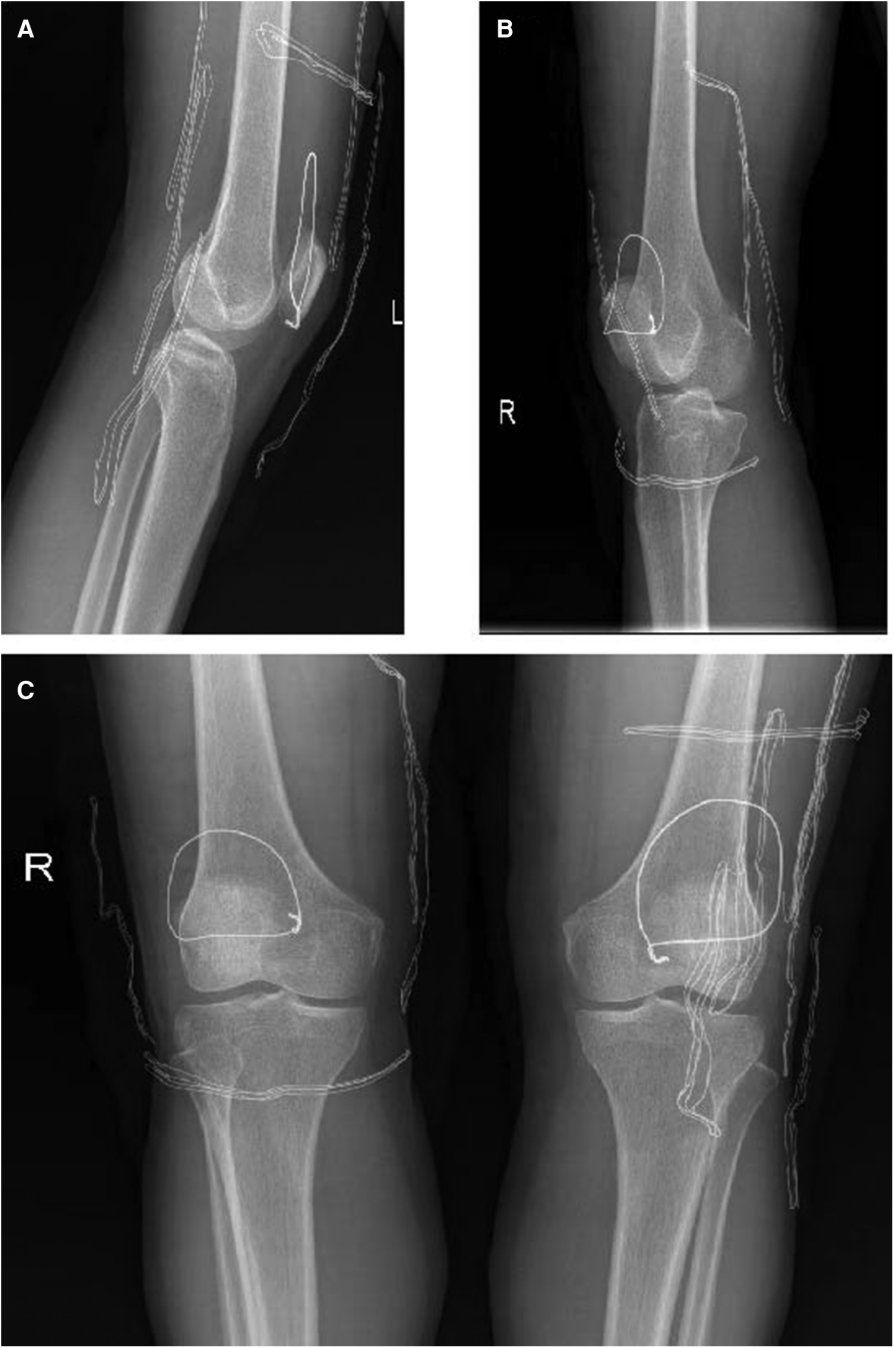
On the first day after operation, x-ray films of both knee joints were re-examined. (A) Lateral x-ray of the left knee; (B) Lateral x-ray of the right knee; (C) Lateral x-ray of the left knee.
